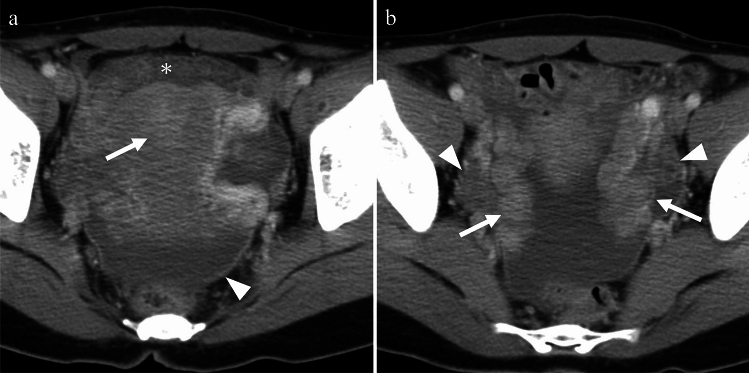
Woman in her 40 s with lower abdominal pain who tested positive for Chlamydia. a Contrast-enhanced CT revealing endometrial enhancement (arrow), indicating potential endometritis. Stranding of the anterior pelvic fat planes (asterisk) and the presence of complex free fluid, and peritoneal thickening (arrowhead) in the dependent areas are also noted. b Bilateral tubal thickening exceeding 5 mm (arrows) on contrast-enhanced CT is consistent with salpingitis. The ovaries exhibit a polycystic appearance (arrowheads) with mild haziness and hyperemia, suggestive of oophoritis
